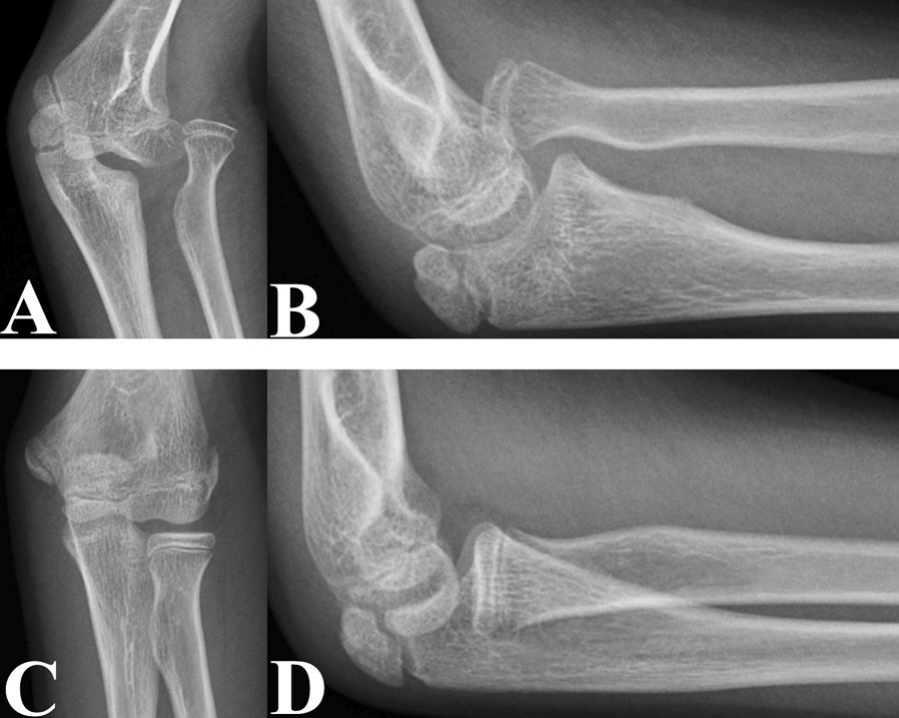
Anteroposterior (A and C) and lateral (B and D) radiographs of the injured (A and B) and uninjured (C and D) elbows in a 12-year-old boy with an untreated CMF, a time from injury to diagnosis of 25 months, and a DD-RH of 2.33%. Lateral radiographs showed a greater En-RH_L_ in the injured elbow (B) than in the uninjured elbow (D)
